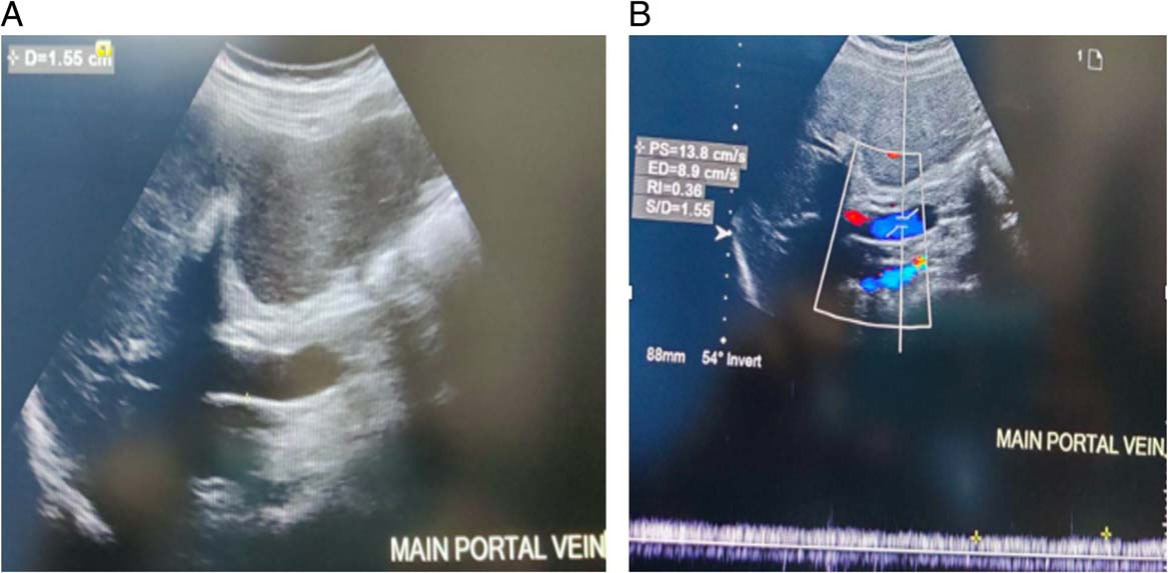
A: Greyscale ultrasound image showing the measurement of the portal vein diameter. B: Spectral Doppler ultrasound image showing maximum and minimum portal vein velocity measurement.
